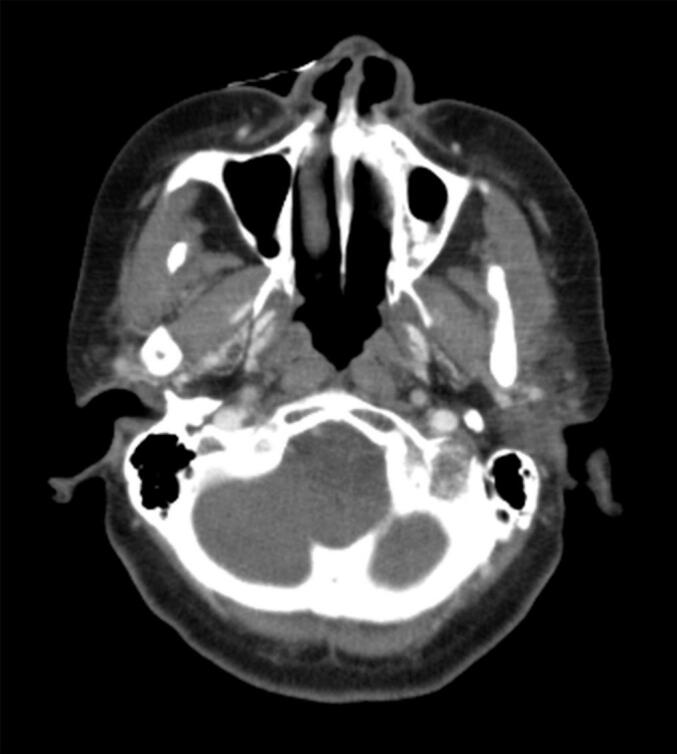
Post-treatment imaging findings CT scan performed 4 months after the initiation of LP therapy shows no detectable lesion in the left mandible, consistent with complete response.
